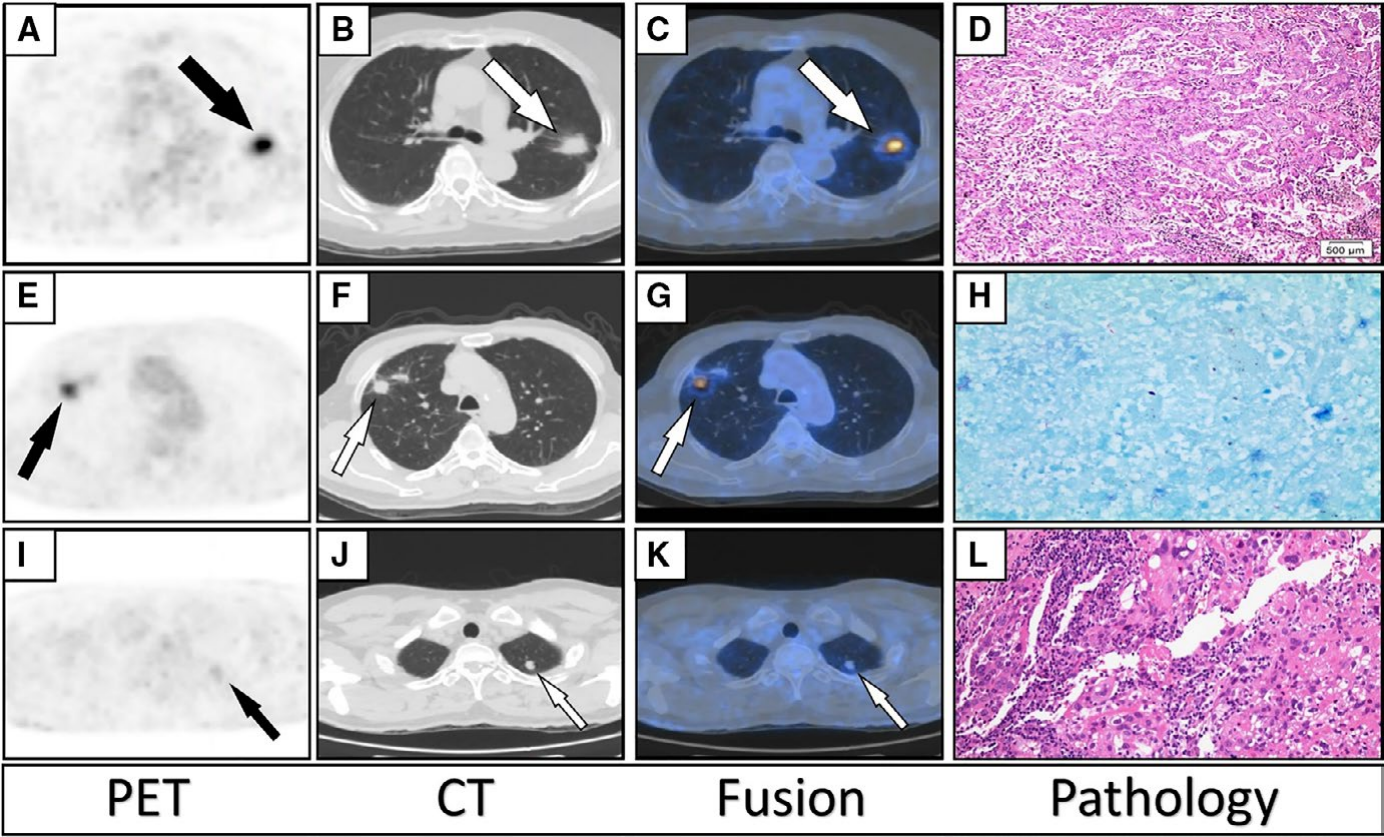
A–D, axial PET, CT, fused PET/CT, and pathology images of a 68 year‐old‐female patient diagnosed with adenocarcinoma on ^18^F‐FDG PET/CT in the upper lobe of the left lung, confirmed by pathology analysis resulting in true‐positive lung cancer; SUVmax:17.5, SA: 2 cm and LA: 2.3 cm. E–H, axial PET, CT, fused PET/CT and pathology images of an 8‐ year‐old male patient diagnosed with lung cancer on ^18^F‐FDG PET/CT in the upper lobe of the right lung but showing PTB on pathology analysis (false‐positive lung cancer); SUVmax: 6, SA: 1.6 cm, LA: 2.1 cm. I–L, axial PET, CT, fused and pathology images of 48‐year‐old male patient diagnosed with PTB on ^18^F‐FDG PET/CT in the upper lobe of the left lung with pathology findings of adenocarcinoma of the lung (false‐negative lung cancer); SUVmax: 2, SA: 0.8 cm, LA: 1.2 cm. (Abbreviations: PET/CT, Positron Emission Tomography/Computed Tomography; FDG, Fluorine‐2‐Deoxy‐ D‐glucose; PTB, Pulmonary tuberculosis; SUVmax, maximum Standardized Uptake value; SA, Short Axis; LA, Long axis)
